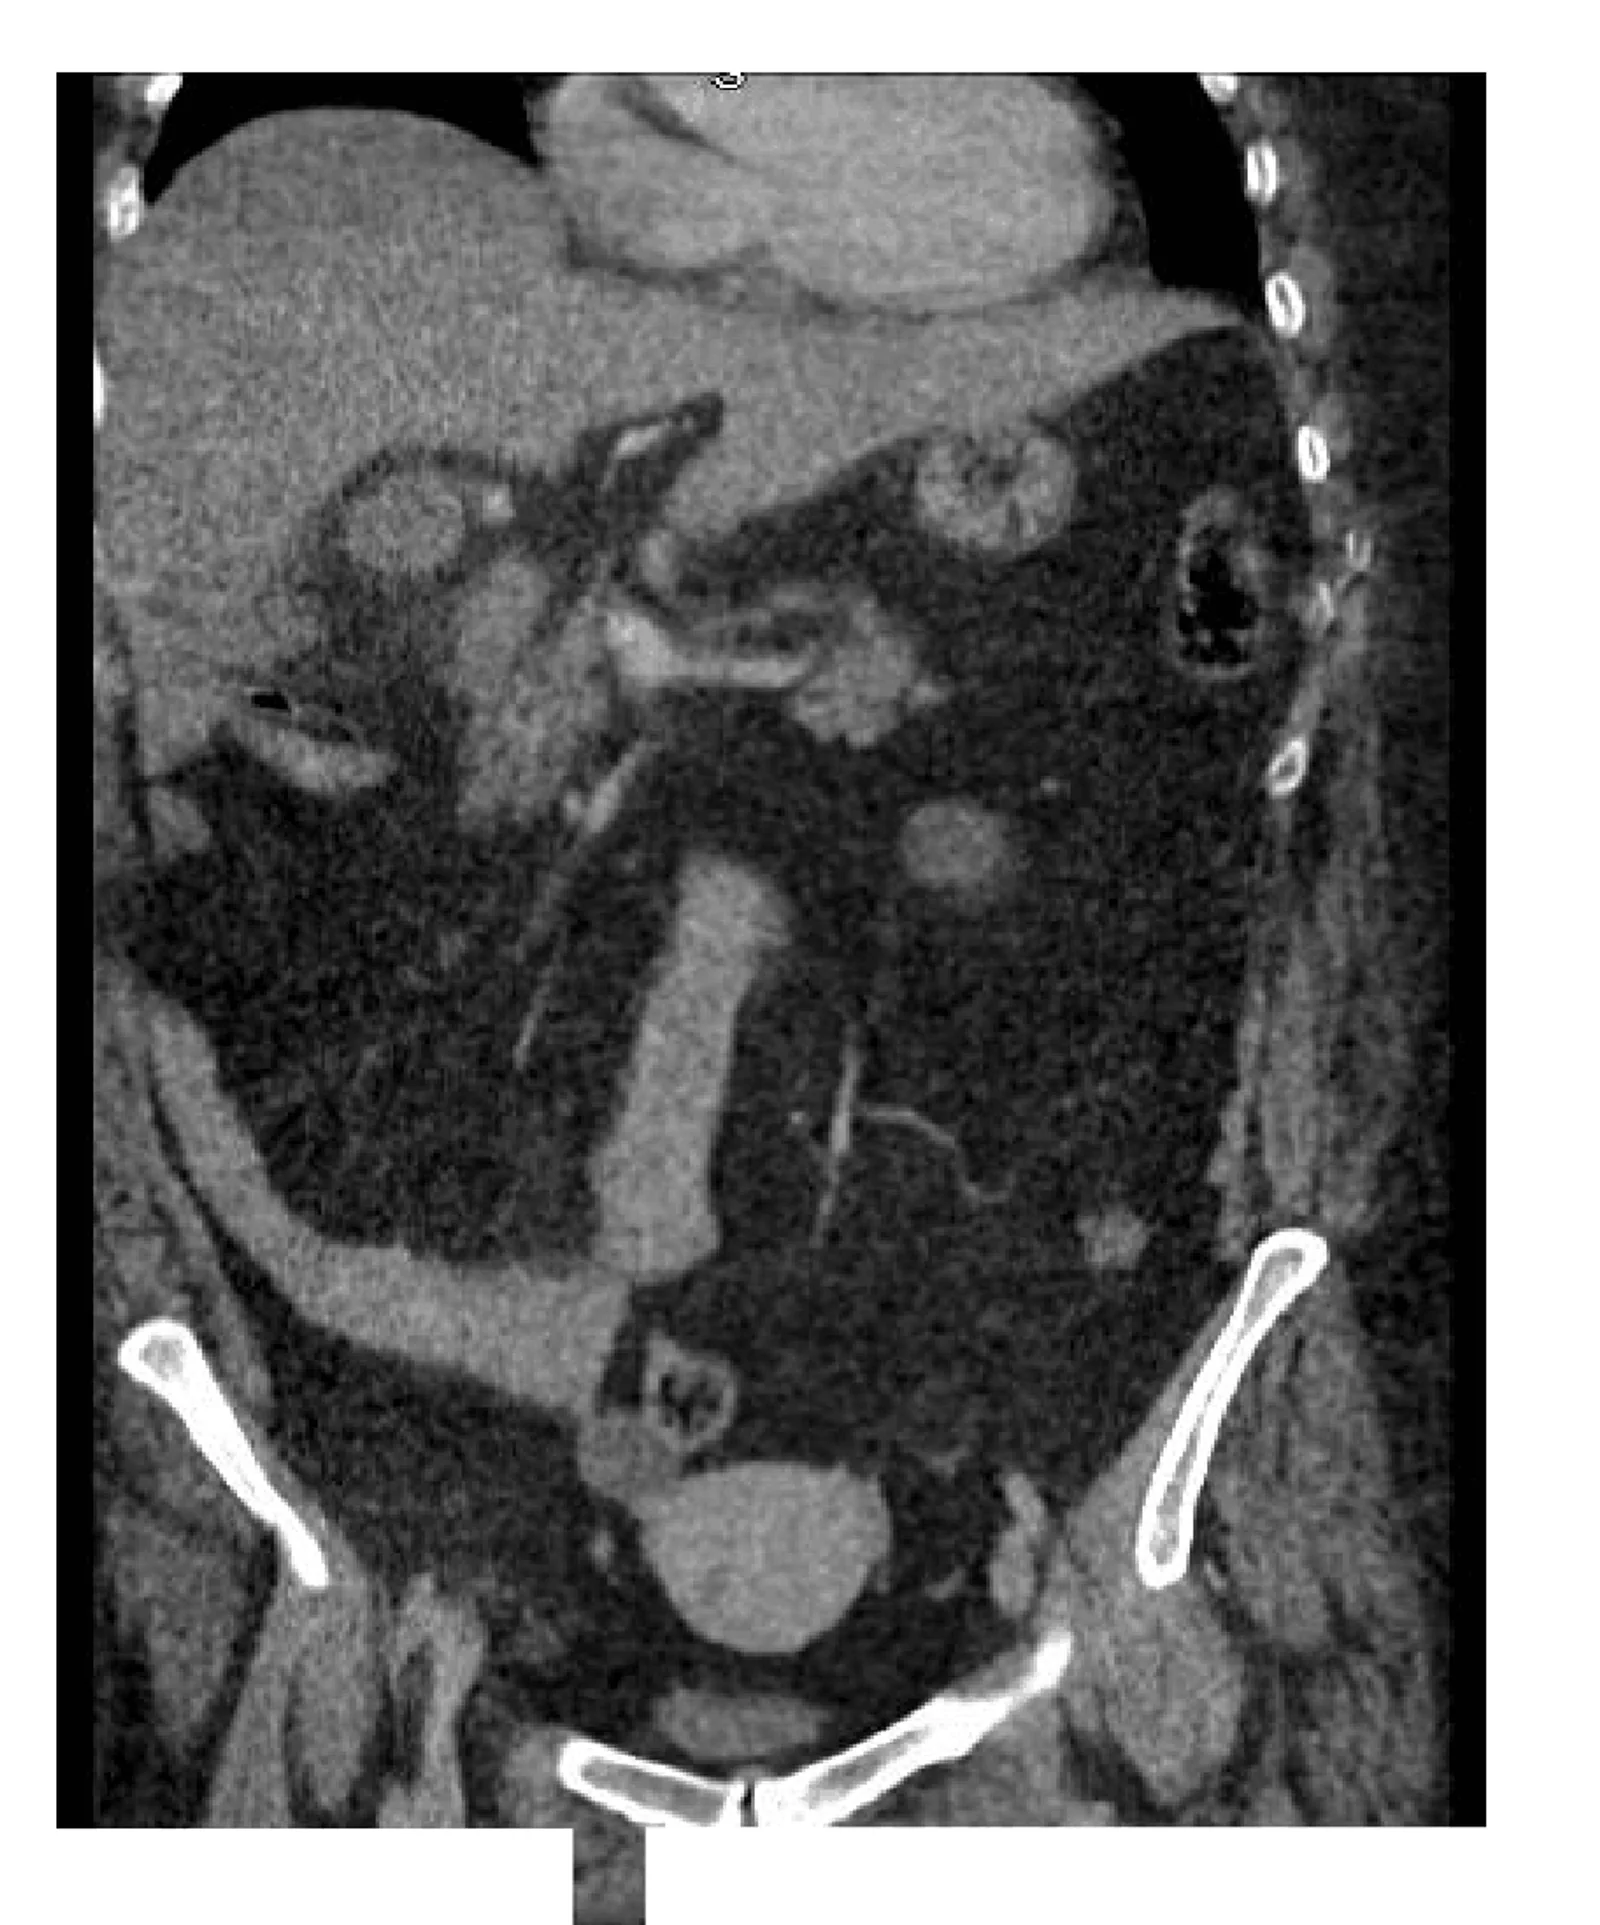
CT coronal section image showing the caecum in the midline and the small bowel on the right side
